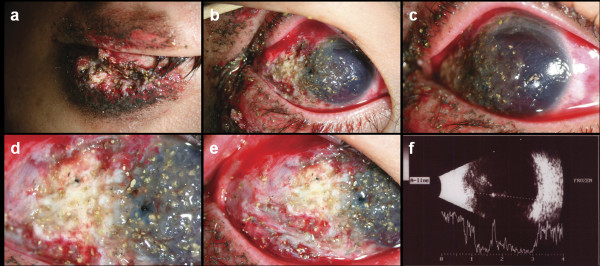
Anterior segment photography and B-scan ultrasonographic findings of patient. a, External photography showed a flame burn of the right upper and lower lid with multiple missile wounds of lids. b-e, Slit lamp examination revealed numerous yellow, powder-like foreign bodies impacted into the ocular surface, severe stromal edema with Descemet's membrane folding, anterior chamber bleeding, lateral rectus muscle splitting injury and traumatic cataract in the right eye. f, Transverse view of B-scan ultrasonography showed hyperechoic foreign bodies with mild reduplication echoes and shadowing.
